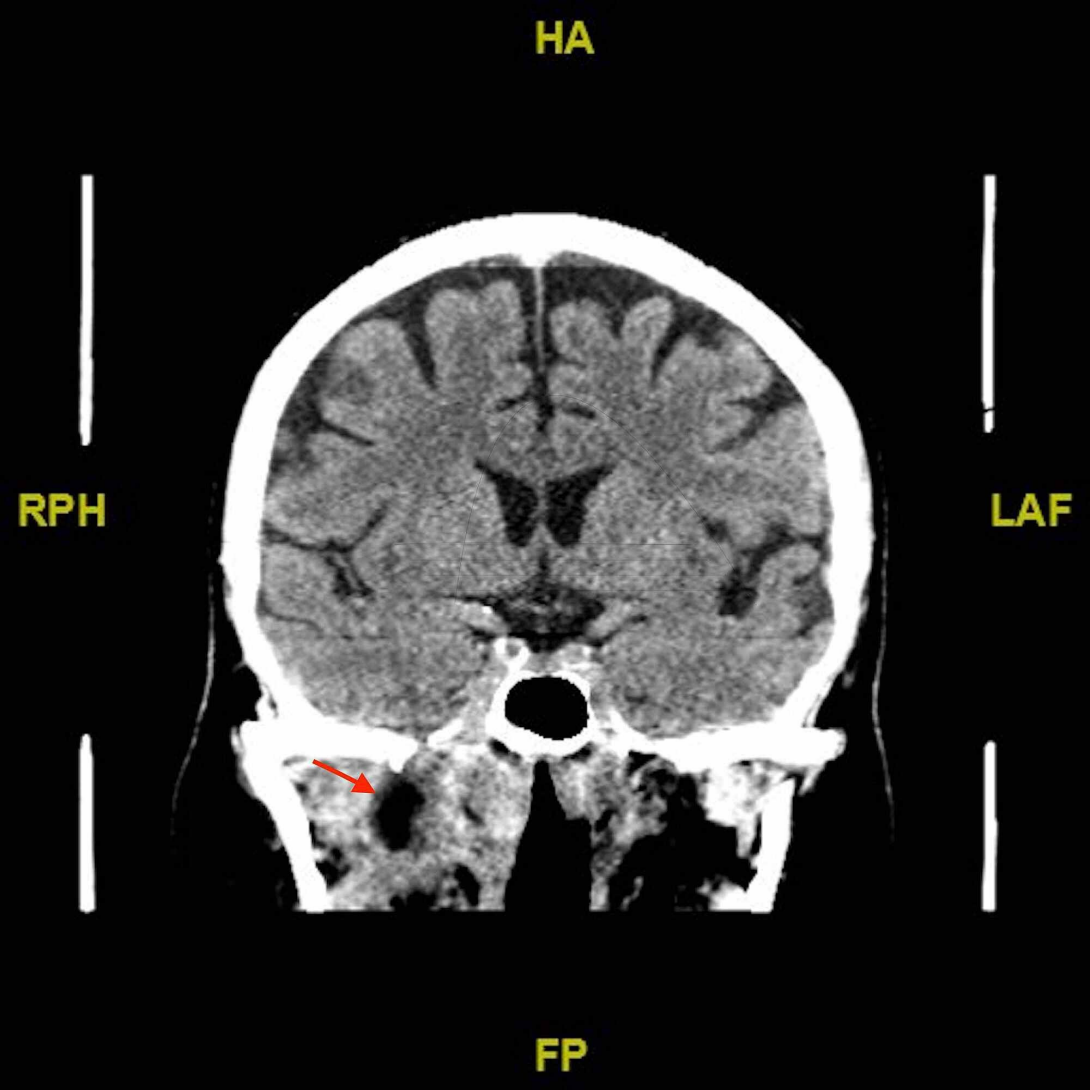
CT scan demonstrating the lesion at the time of presentation CT: computed tomography
